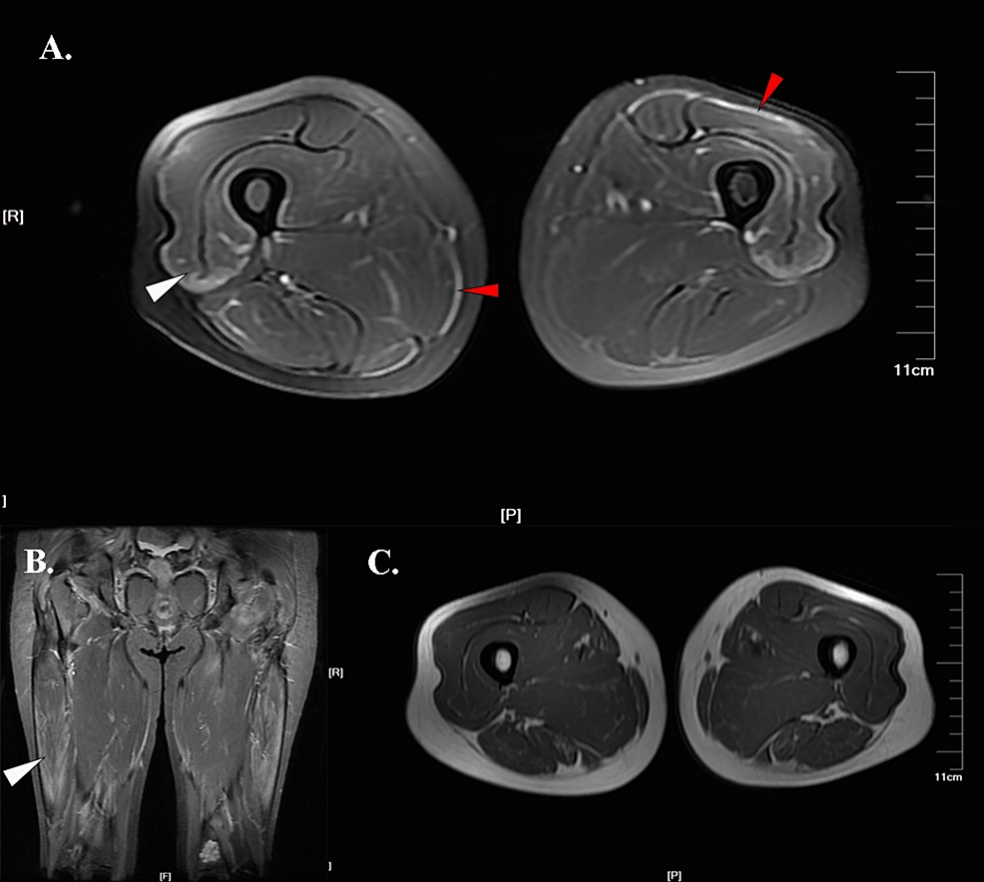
Thigh muscle MRI images of Case 3 A. An axial T2 fs sequence image showed fascia involvement (red arrows) and peripheral honeycomb edema signal of the vastus lateralis (white arrow). B. A coronal STIR sequence image showed a vastus lateralis edema signal (white arrow). C. An axial T1 sequence image showed no fatty infiltration. Abbreviations: T2 fs, T2-weighted fat saturation; STIR, short time of inversion recovery
